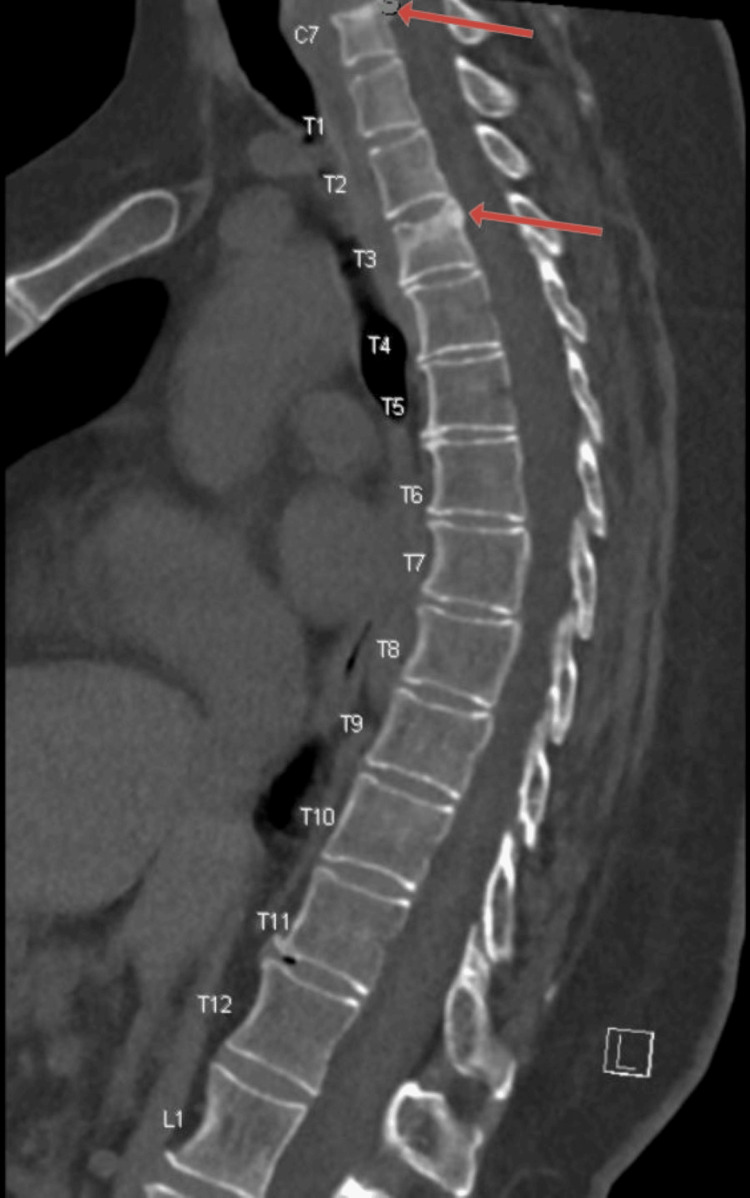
CT thoracic spine without IV contrast Demonstrating a subacute to a chronic fracture involving the superior endplate of C7 and an acute to subacute superior endplate fracture involving T3 (red arrows)
